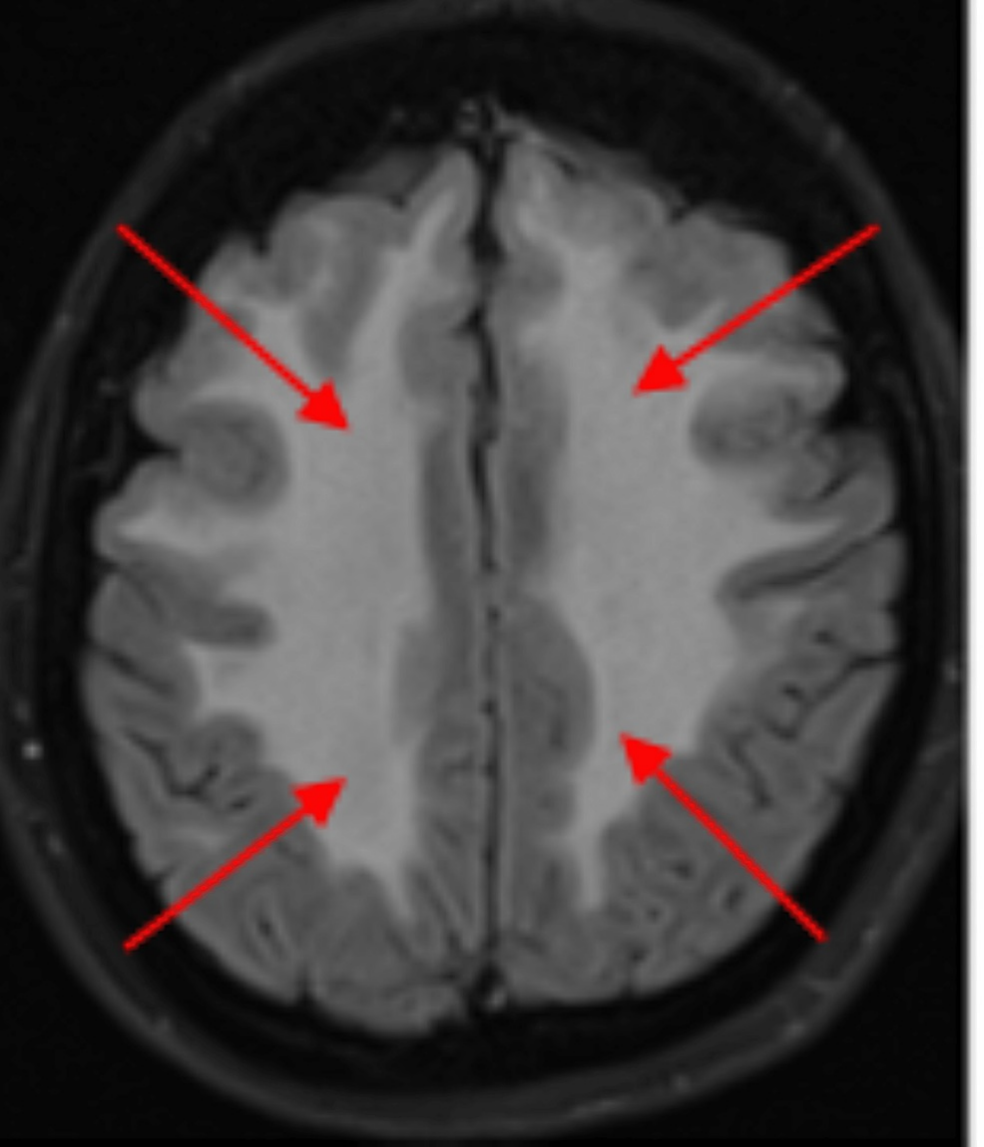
MRI FLAIR sequence showing extensive white matter signal abnormality (red arrows). FLAIR: fluid-attenuated inversion recovery.
